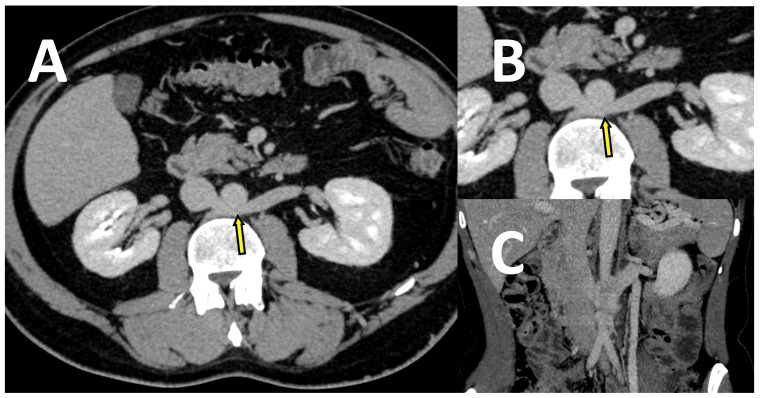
The images of a CT-V scan in a patient with a retroaortic location of LRV. LRV (marked with arrow) is compressed between the aorta and corpus of the lumbar vertebra, as shown on the transverse plane of whole (A) and enlarged image (B), or on the coronal plane (C).
